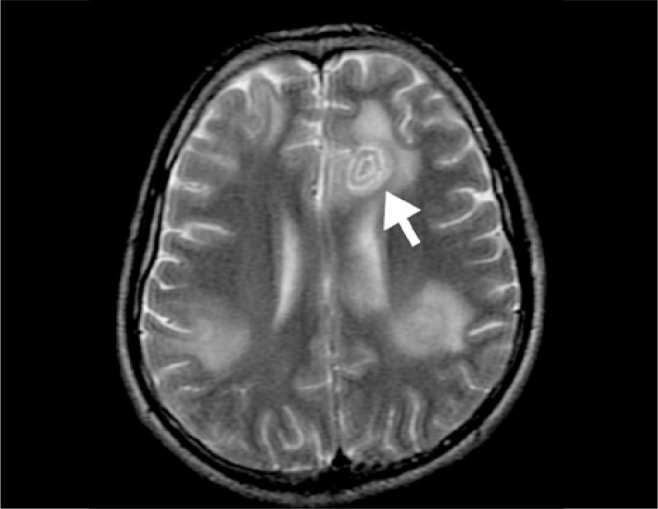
MRI of a 25-year-old male patient with AIDS, after an ini-tial
